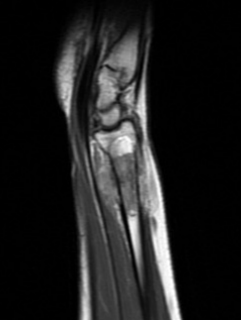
Sagital T1W MR image, after contrast medium injection. Medullary and soft tissue extensions are well evaluated.
